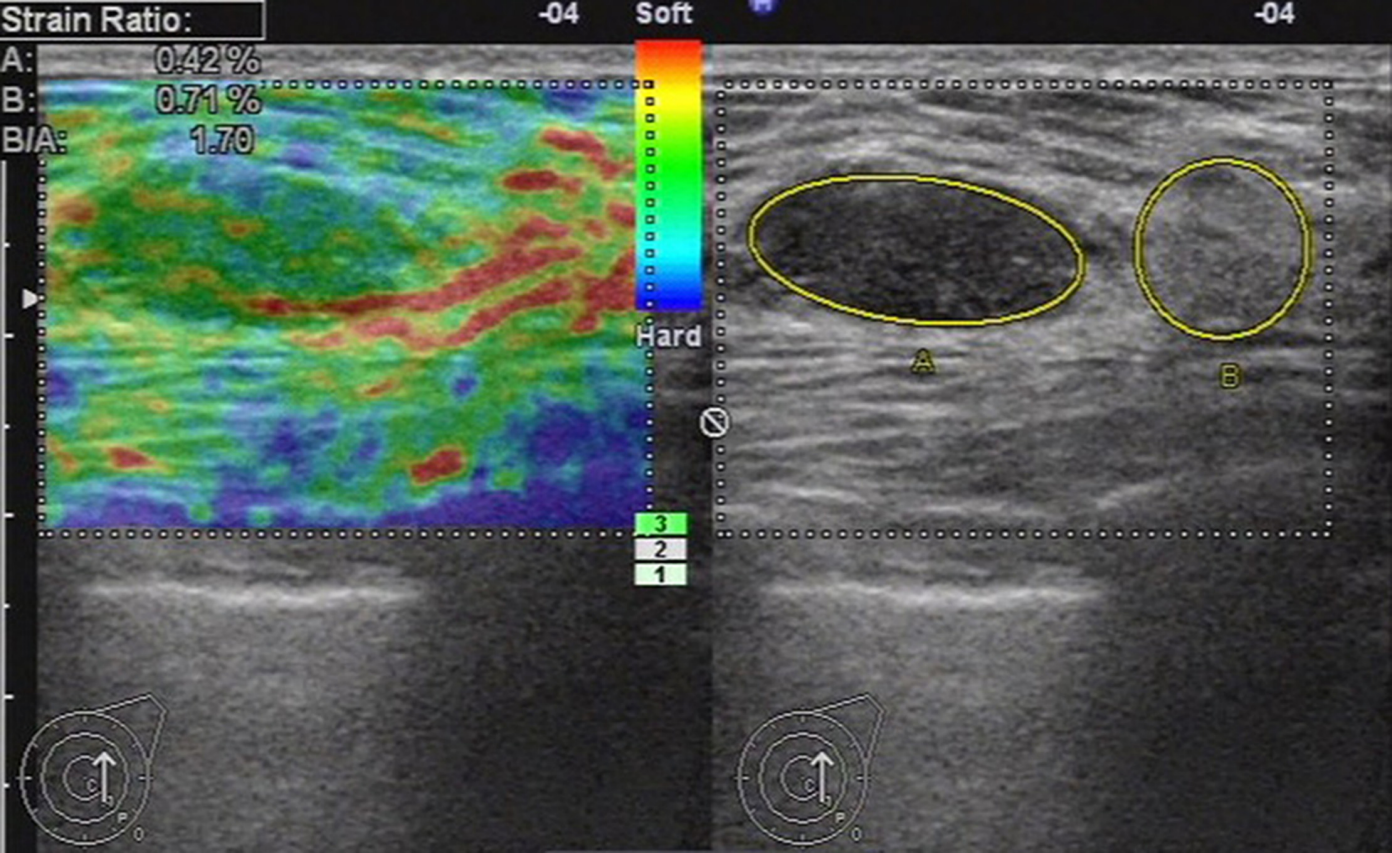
Ultrasonic elastographic image of a fibroadenoma in a 38-year-old woman. The lesion was scored 2 with the 5-point scoring method. The strain ratio was 1.70 with the strain ratio method at the same breast tissue depth as the reference.
